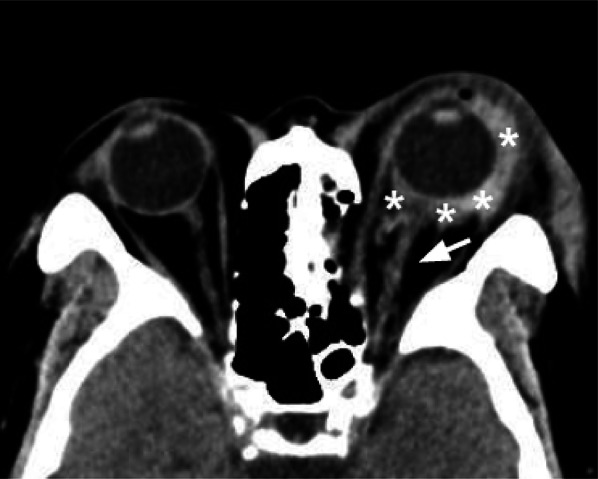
Axial CT acquisition reconstructed with soft tissue algorithm in an orbital blunt trauma with fracture of the left lamina papyracea, in a patient who complained left visual loss. The left optic nerve is stretched, mildly swollen, and hyperdense (white arrow). These findings are suggestive for post-traumatic optic neuropathy. The globe is surrounded by a hyperdense hematoma (asterisks), extended in the retro-orbital fat tissue next to the optic nerve
